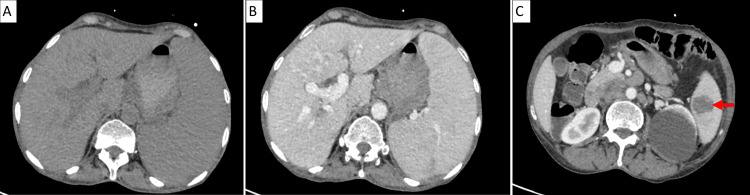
Abdominal sections from the CTCAP showing splenomegaly with a lower pole splenic tissue lesion CTCAP: chest-abdomen-pelvis computed tomographic scan (A) Transverse section without contrast. (B) Transverse section with contrast. (C) Transverse section with contrast centered on the lower pole tissue lesion (arrow)
